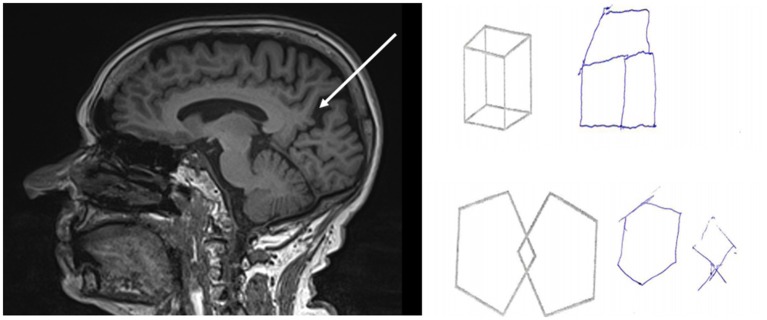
Results of brain MRI and neuropsychology testing of a mild Alzheimer patient with epileptic seizures. Figure demonstrates the impaired visuo-spatial skills examined with Addenbrooke Cognitive Examination and visible precuneal degeneration on MRI with deep sulci surrounding the precuneus (white arrow).
